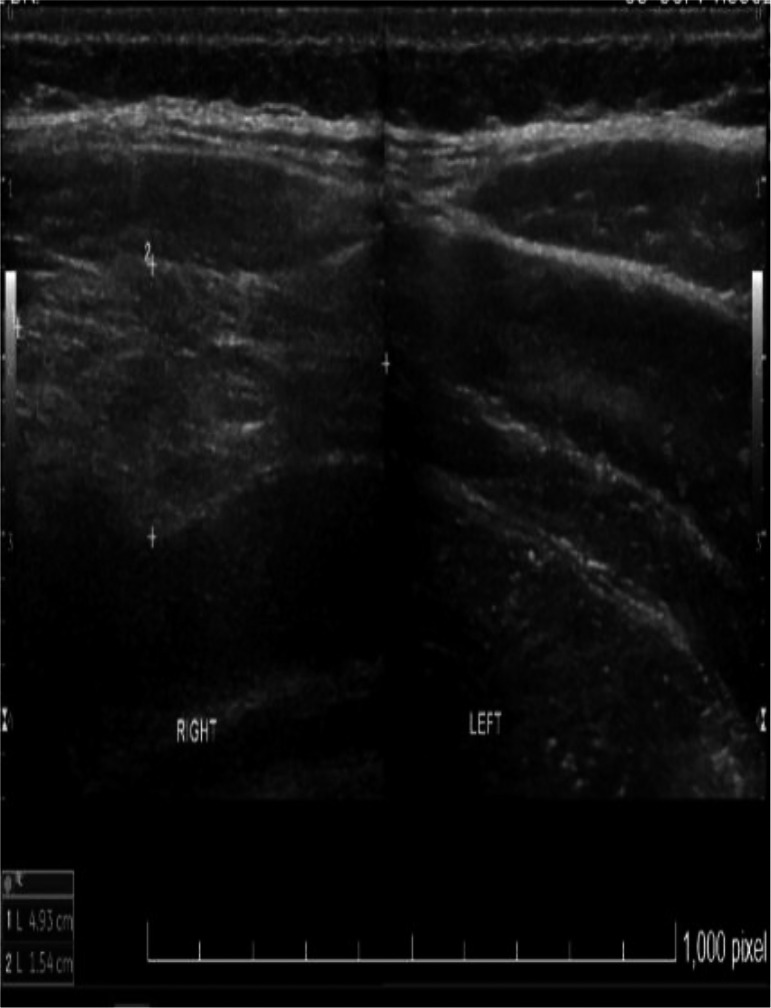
Ultrasound of soft tissue showing a large echogenic solid lesion in the anterior abdominal wall on the right side between the abdominal wall muscles measuring approximately 10 × 8 × 2.4 cm
